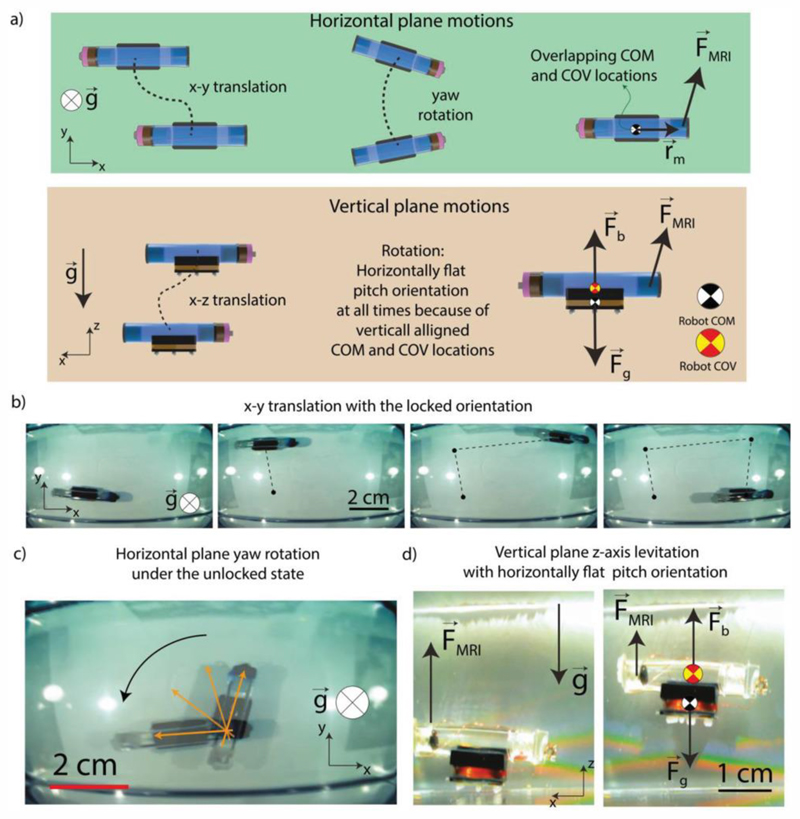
Motion degrees-of-freedom of the REVOLBOT. a) On the horizontal plane, REVOLBOT can translate along the *x*- and *y*-axis. REVOLBOT under an unlocked state also has the freedom to rotate and change its yaw orientation actively with the magnetic pulling forces generated by an MRI device. On the vertical plane, the robot can be levitated and moved along the*x*- and *z*-axis, where -*z*-axis is the gravity direction. b) Experimental video snapshots for x and y translation with a fixed orientation. c) Yaw rotation of a robot under the unlocked state. d) Experiment snapshots from Video S1 in the Supporting Information. During the levitation, the robot always stays horizontally flat in the water because of the vertical shift on the position of the robot’s COM location (Text S1 in the Supporting Information).
